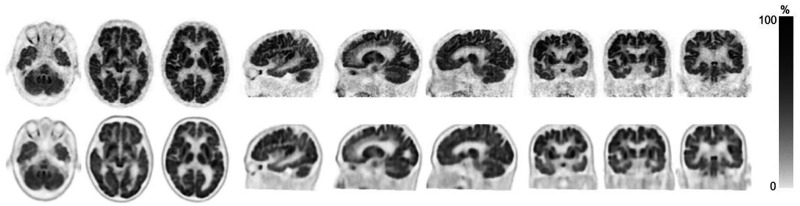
Representative ^18^F-FDG PET image acquired on dhPET scanner (top row) and conventional wbPET scanner (bottom row). Patient is 85-y-old man with mild cognitive impairment due to Alzheimer disease. Bilateral hypometabolism of posterior cingulate gyri, precuneus, and temporal lobe is shown.
